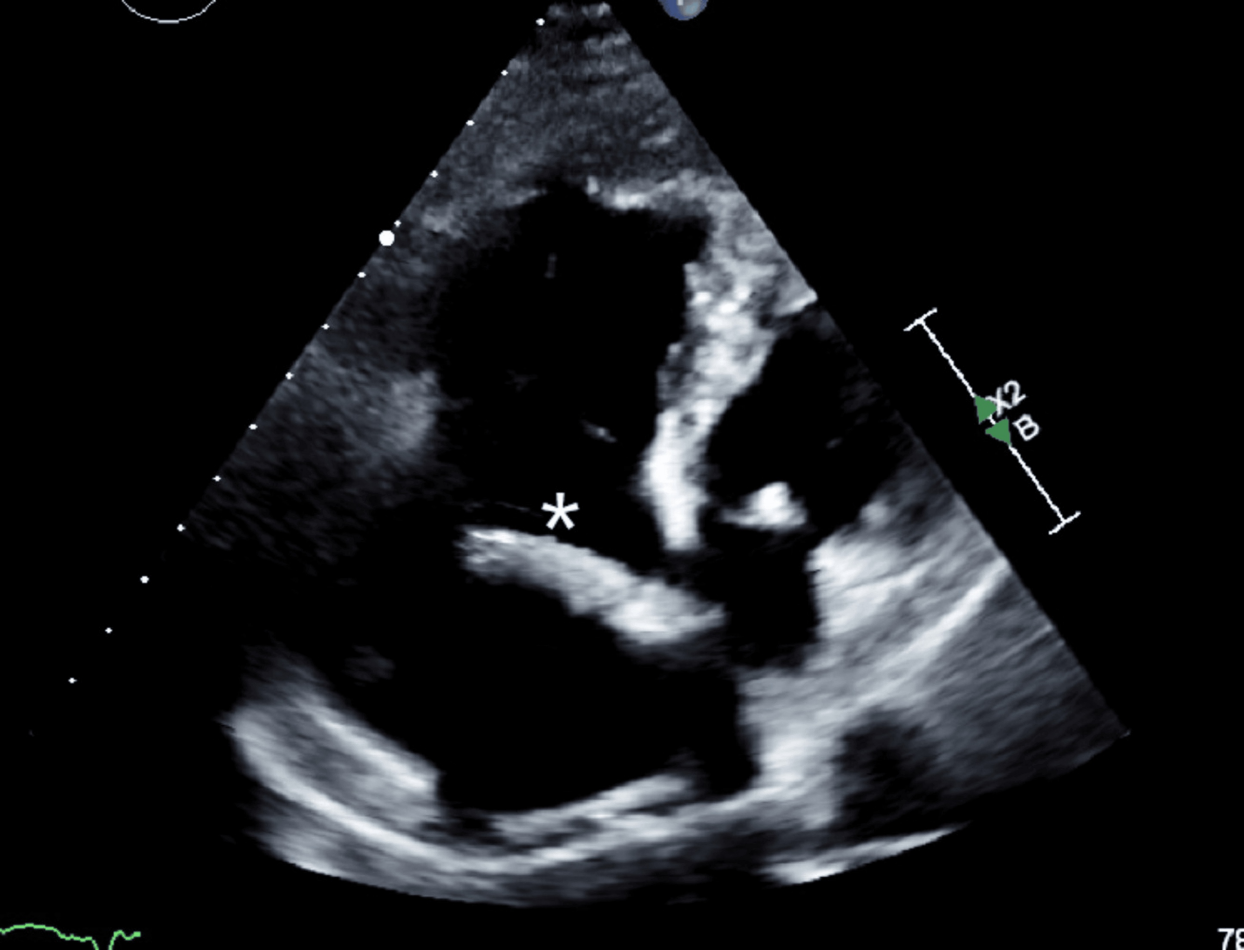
Transesophageal four-chamber view showing an elongated structure (asterisk) originating from the coronary sinus
